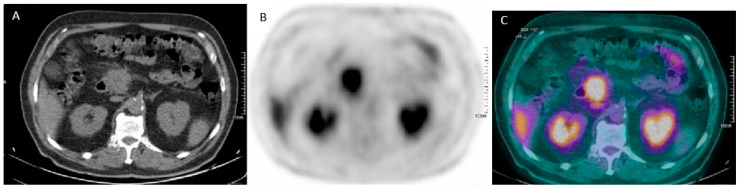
Transaxial low-dose co-registered CT (A), PET (B) and fused PET/CT (C) images show increased ^18^F-FDG uptake in the pancreatic tumoural lesion (C).
